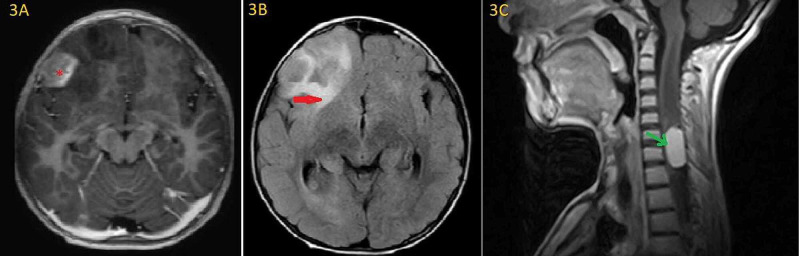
MRI brain and cervical spine. A: Heterogeneously enhancing cortical and subcortical lesions in the right frontal lobe (red asterisk) with associated surrounding vasogenic edema (red arrow) causing complete effacement of the anterior horn of right lateral ventricle (B). C: MRI cervical spine shows homogenously enhancing extra-axial dural-based abnormality in the spinal canal at the level of C7-T2 vertebral bodies suggestive of meningioma (green arrow).
